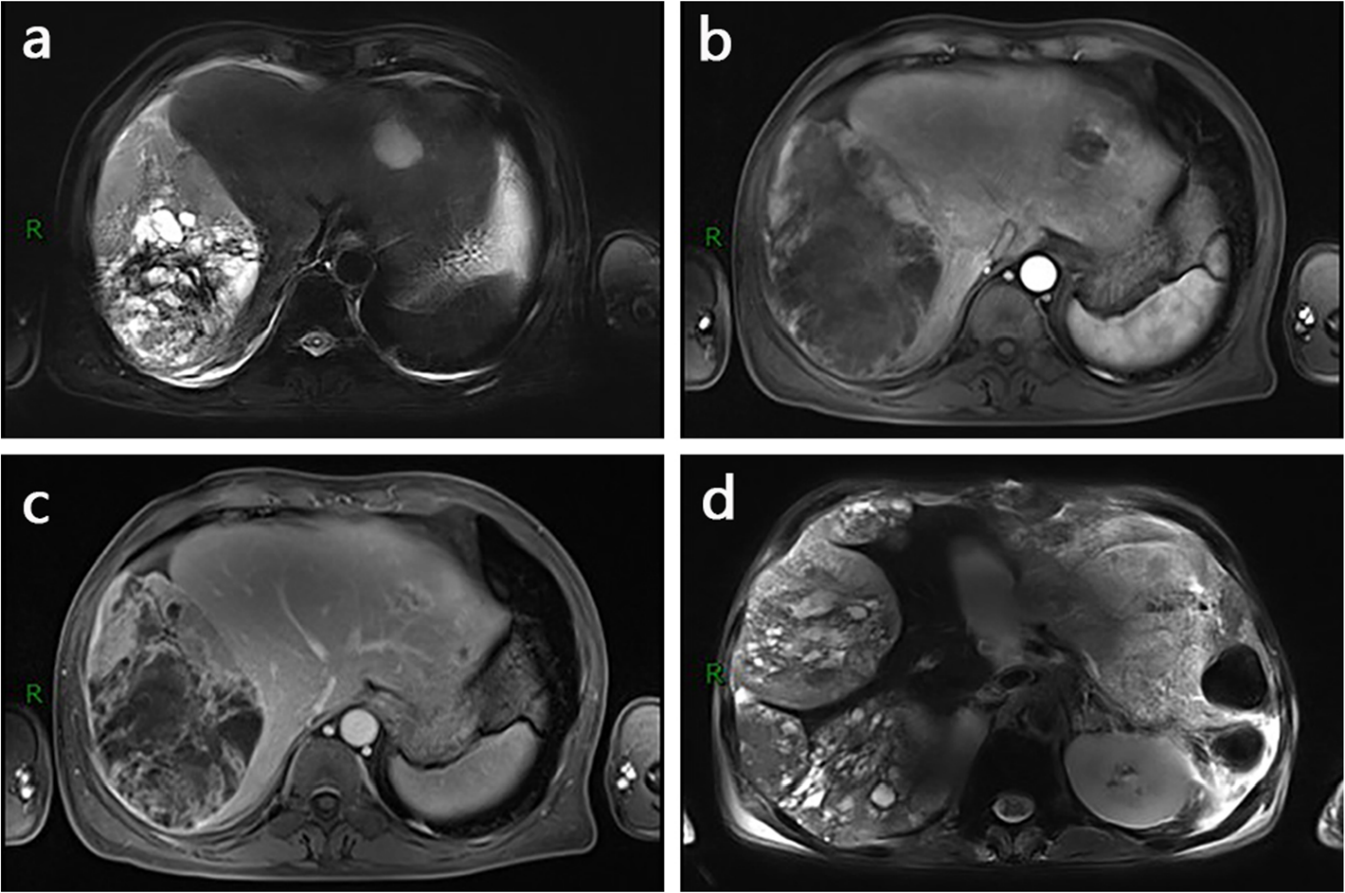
Sarcomatous intrahepatic cholangiocarcinoma in a 55-year old man. T2-weighted TSE BLADE sequence presents a protruding bulging mass with multilocular cyst-like changes and hemorrhage (a). Dynamic gadoxetic acid–enhanced MR images show hyper irregular peripheral enhancement on the AP (b) followed by peripheral wash out and centrally progressive enhancement with septa on the later phase (c). The mass recurs one month after surgery (d)
